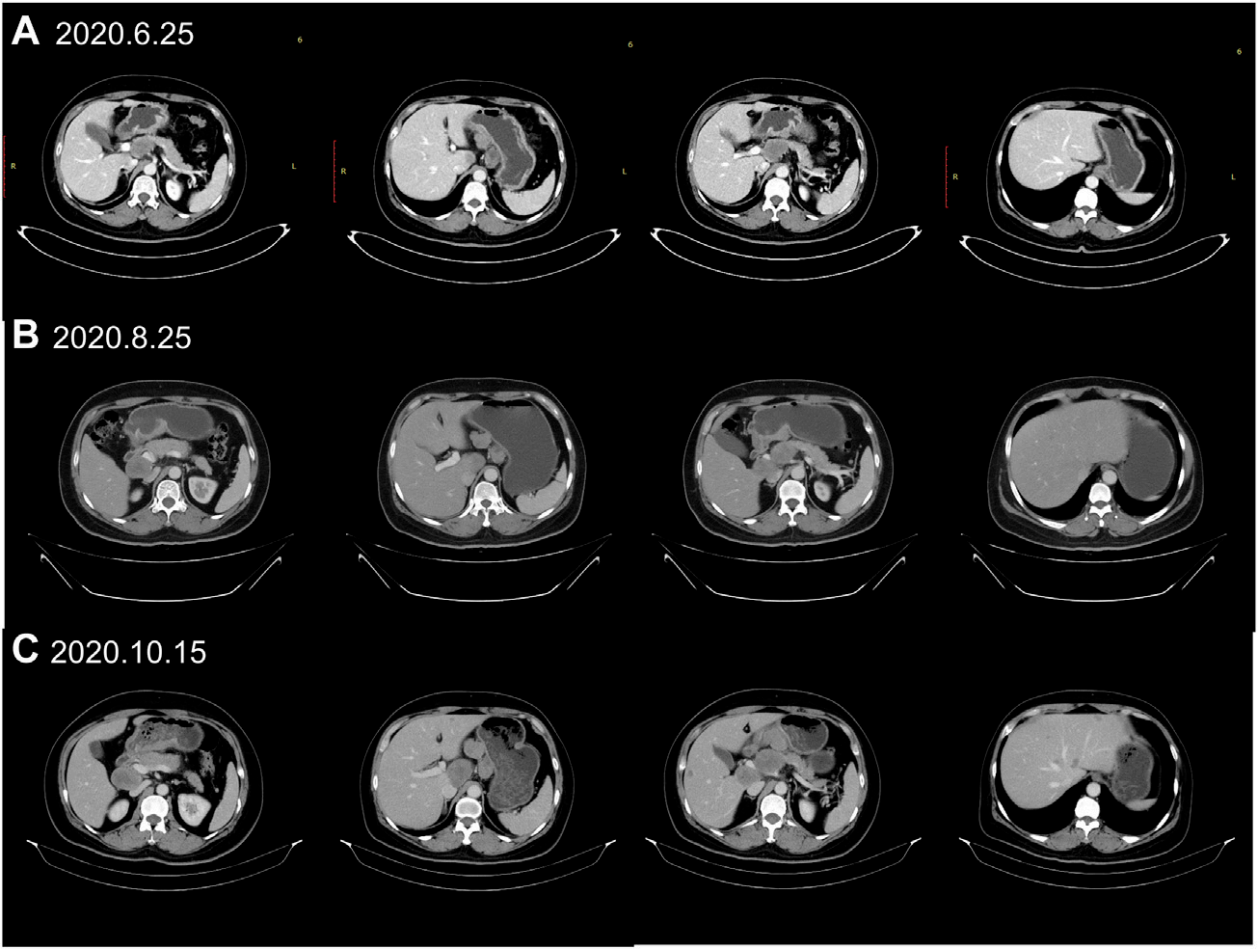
Treatment protocol before trastuzumab therapy. (A) CT scans (2020.6.25) showed the patient’s baseline disease; (B) CT scans (2020.8.25) demonstrated progressive disease (PD) in multiple lymph nodes metastases when first-line chemotherapy failed. (C) CT scans (2020.10.15) revealed new liver metastases when the second-line chemotherapy failed.
